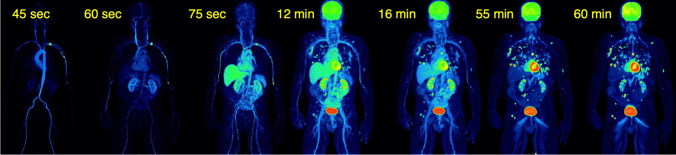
Dynamic [18F]-FDG PET-CT study in a patient with metastatic melanoma. Serial torso images from 45 s to 60 min p.i. demonstrate the high temporal resolution of the LAFOV scanner in the first seconds of dPET acquisition with the delineation of the large arterial vessels as well the gradual increase of the tracer uptake in the tumor lesions up to 60 min. PET angiography based on early dynamic PET-CT images may become an option for vascular evaluation, given the satisfying spatial and temporal resolution of the LAFOV systems
